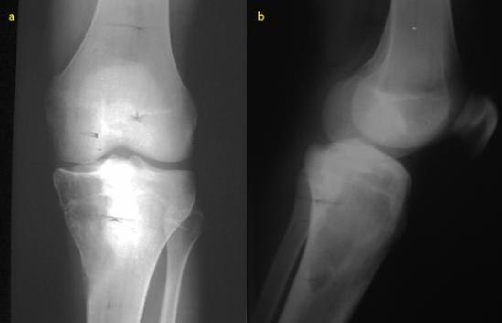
Plain X-ray examination of LT knee (anteroposterior and lateral view).
